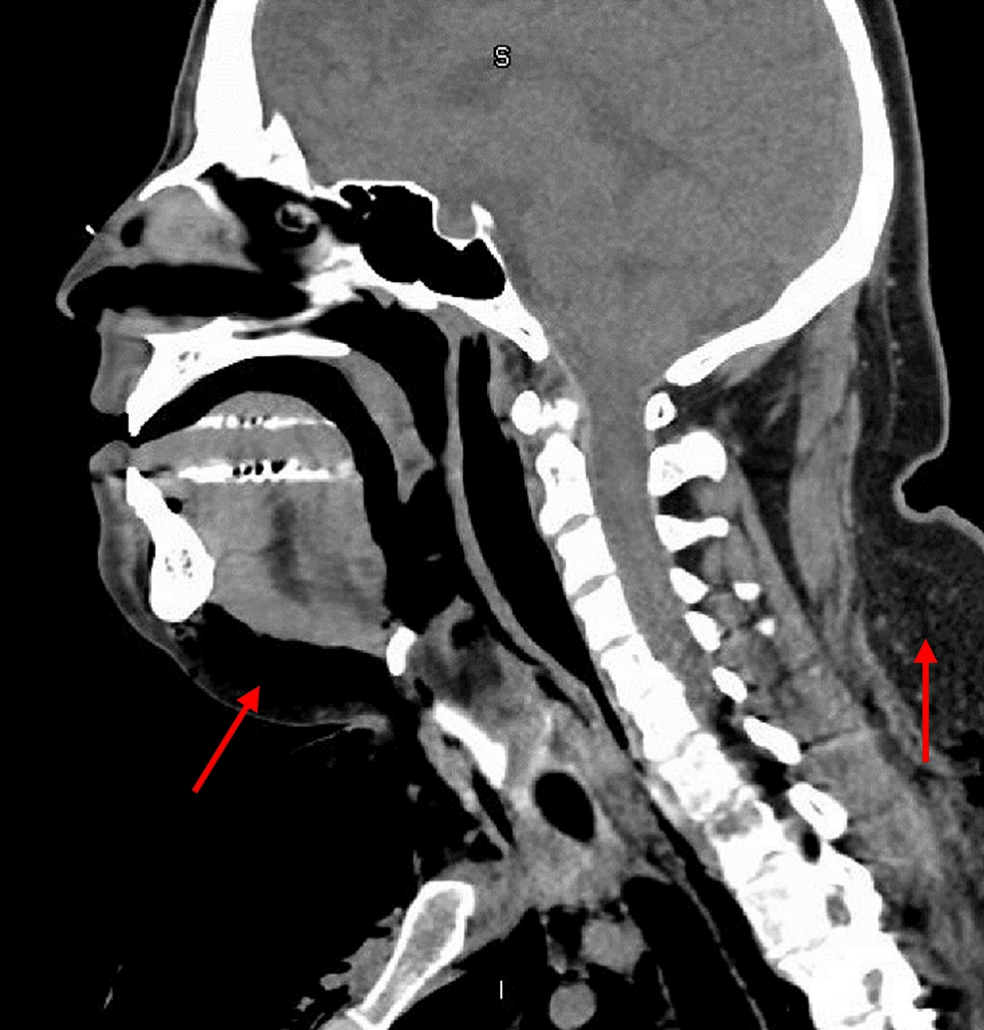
Sagittal view of CT neck indicating the extension of subcutaneous emphysema (red arrows).
